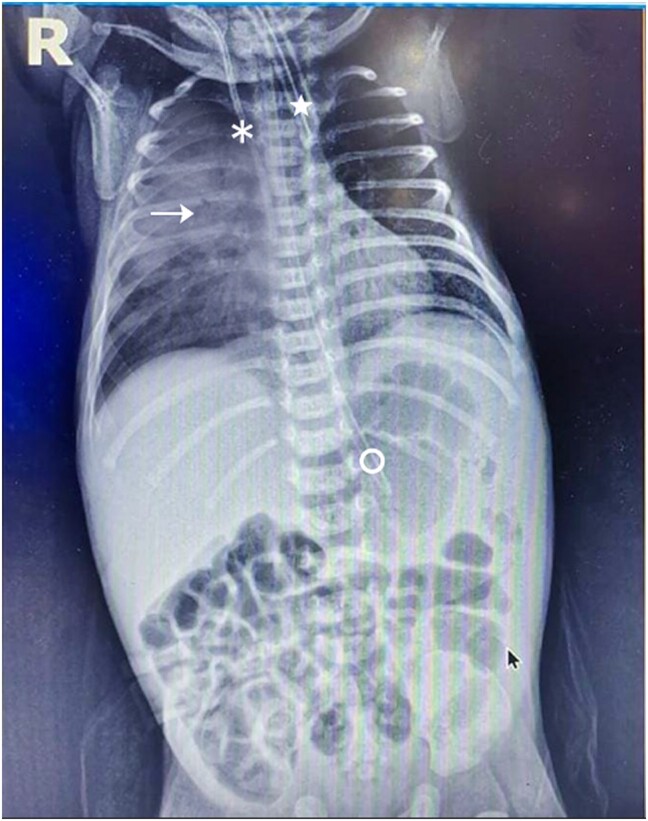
Chest X-ray showing right central venous catheter (asterisk), endotracheal tube (star), nasogastric tube (circle) and right lung consolidation (arrow).
